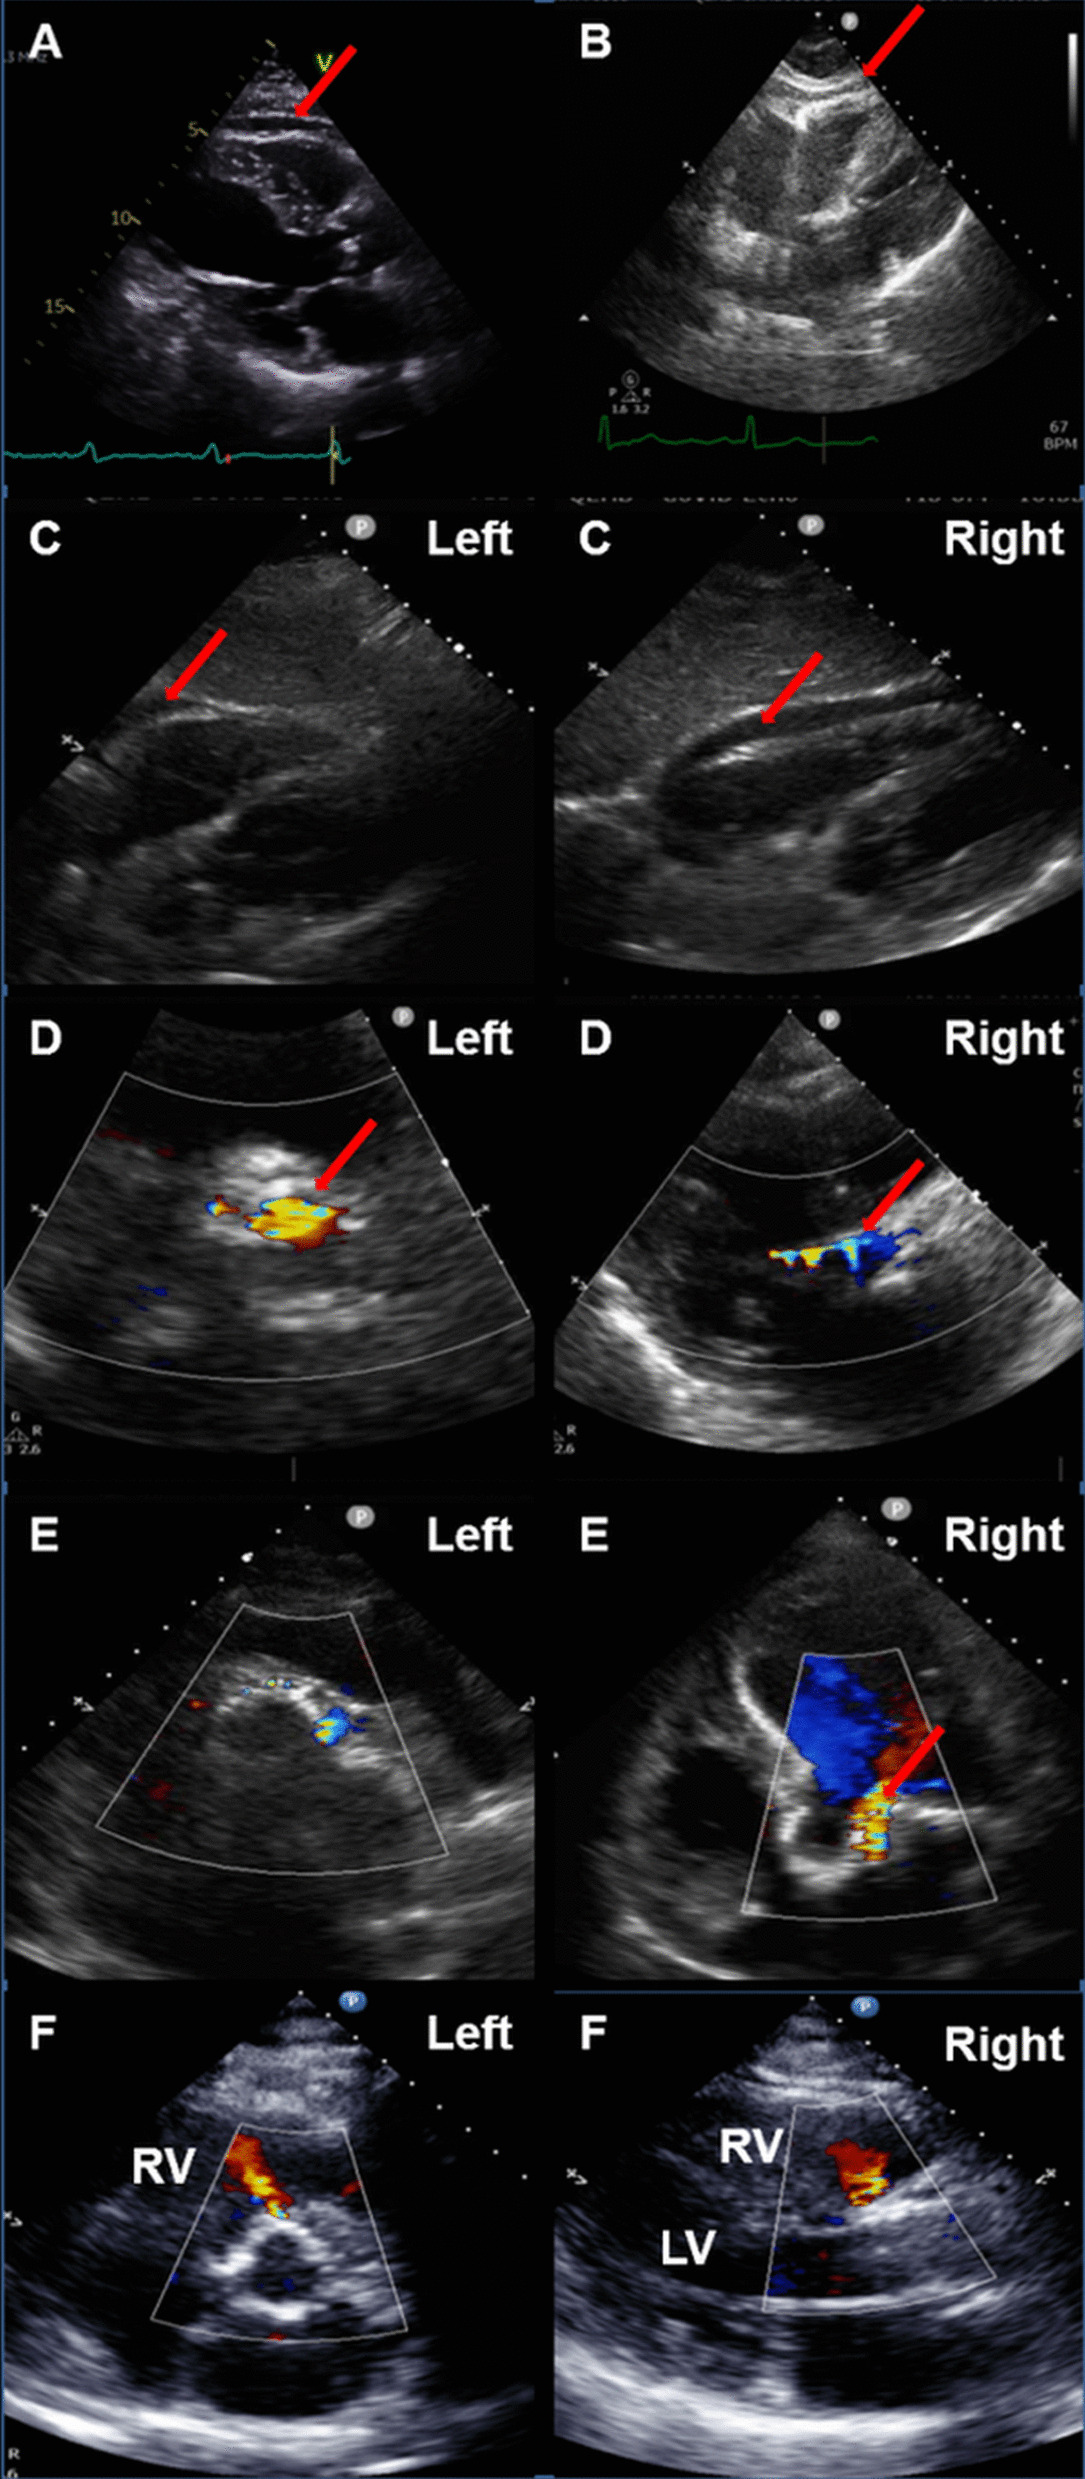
A Baseline echocardiogram showing small < 1 cm pericardial effusion (arrow). B A pericardial fat pad was noted on the baseline echocardiogram (arrow). This may be confused with a pericardial effusion on the post-deployment study. C A small pericardial effusion (arrow) was noted on the baseline study (Left). Post THV deployment the effusion enlarged (arrow) and required emergency pericardiocentesis (Right). D Long axis view showing wire-induced AR (arrow). The colour Doppler jet often ‘hugs’ the wire (Right). On the short axis view the wire-induced AR lies within the frame (Left) and is adjacent to the stiff wire (arrow). E On short axis a moderate jet of PVR can be seen at 3 o’clock with trivial jets at 10–12 o’clock. The jets lie outside the THV frame (Left). Apical 5 chamber view showing the PVR jet (arrow) outside the frame (Right). F A interventricular VSD was noted after deployment of a balloon expandable THV. The VSD was visible on the short axis (left) and long axis (right)
